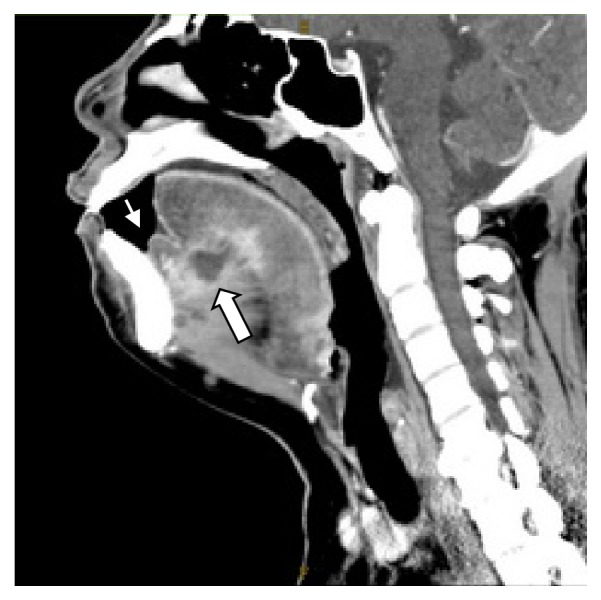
Contrast-enhanced CT scan showed the abscess confined at the ventral aspect of the tongue with sublingual space cellulitis (thick arrow) and marked swelling of anterior floor of mouth was demonstrated (thin arrow).
